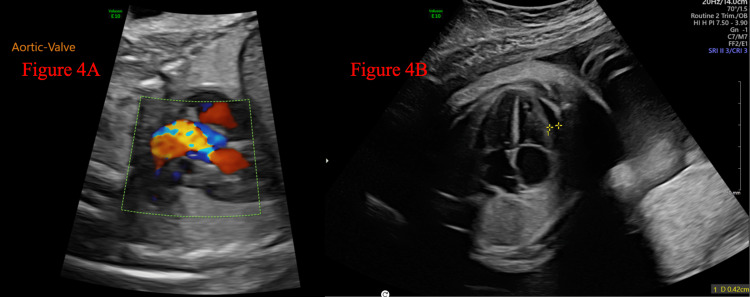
Echocardiogram at 32 and 6/7 weeks of gestation (A) LVOT view demonstrating turbulent blood flow through the narrowed aortic outflow tract. (B) Apical four-chamber view of the fetal heart minimal pericardial effusion measuring 4.2 mm on echocardiography performed at 32 and 6/7 weeks of gestation LVOT: left ventricular outflow tract
